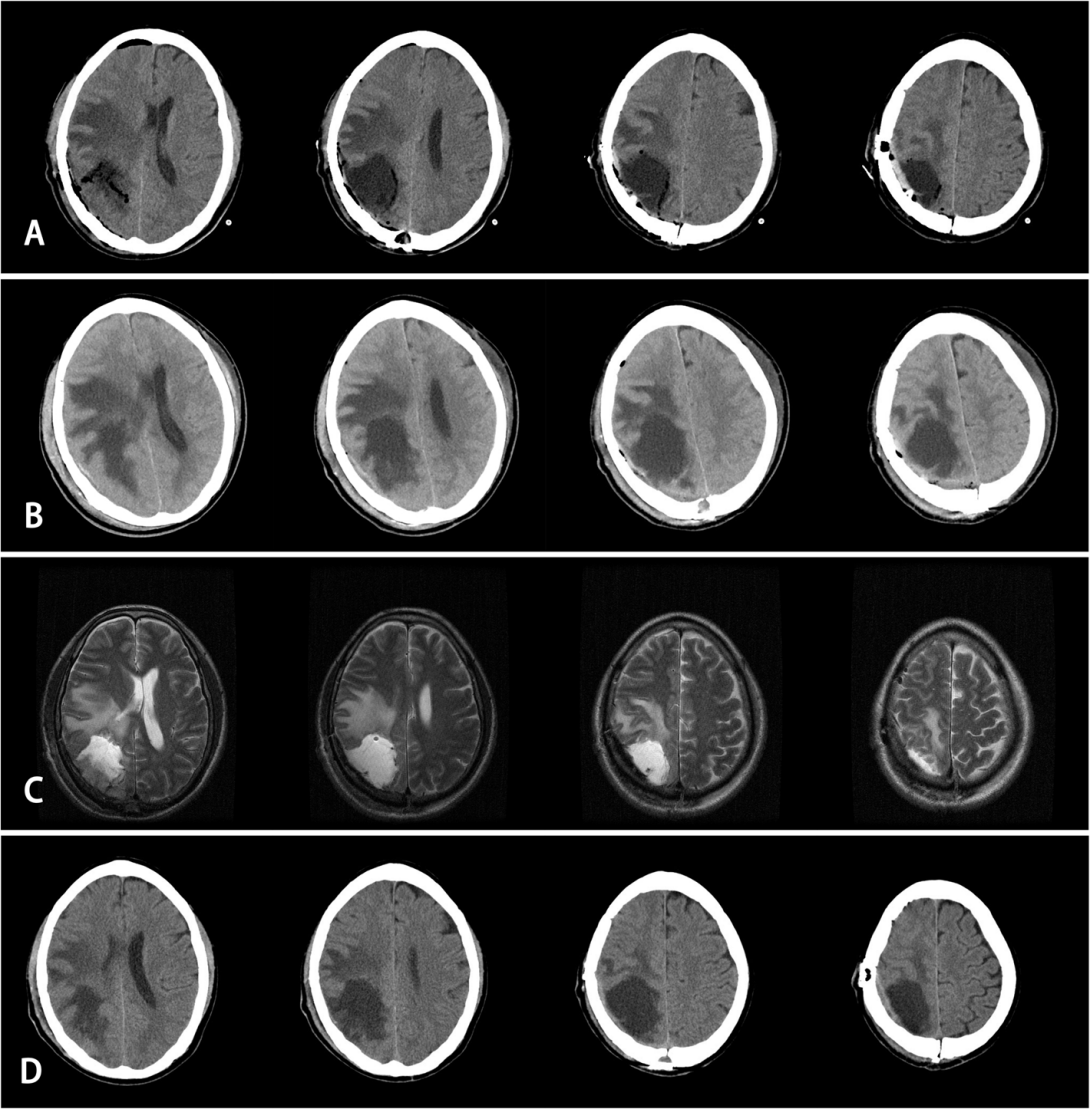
STH occurred in a patient in DG. Because of the watertight suture of the dura, only 10 ml of liquid was drawn out on the 2st POD. So the ED was pulled out. Severe headache occurred from the 3rd day. CT showed swelling of the brain tissue and STH and the mannitol q6h 250 ml was still not efficacious. After 30 ml of liquid from the subdural cavity was extracted via a puncture with a long needle through the bone hole, the symptoms were relieved. Therefore, a subsequent operation did not need to be performed. A CT scans showed light swelling of brain tissue. B CT scans showed severe swelling of brain tissue and STH on the 3rd POD. C MRI showed the swelling of brain tissue was reduced on the 4th POD. D CT scans showed light swelling of brain tissue and no headache suffered on the 10th POD
